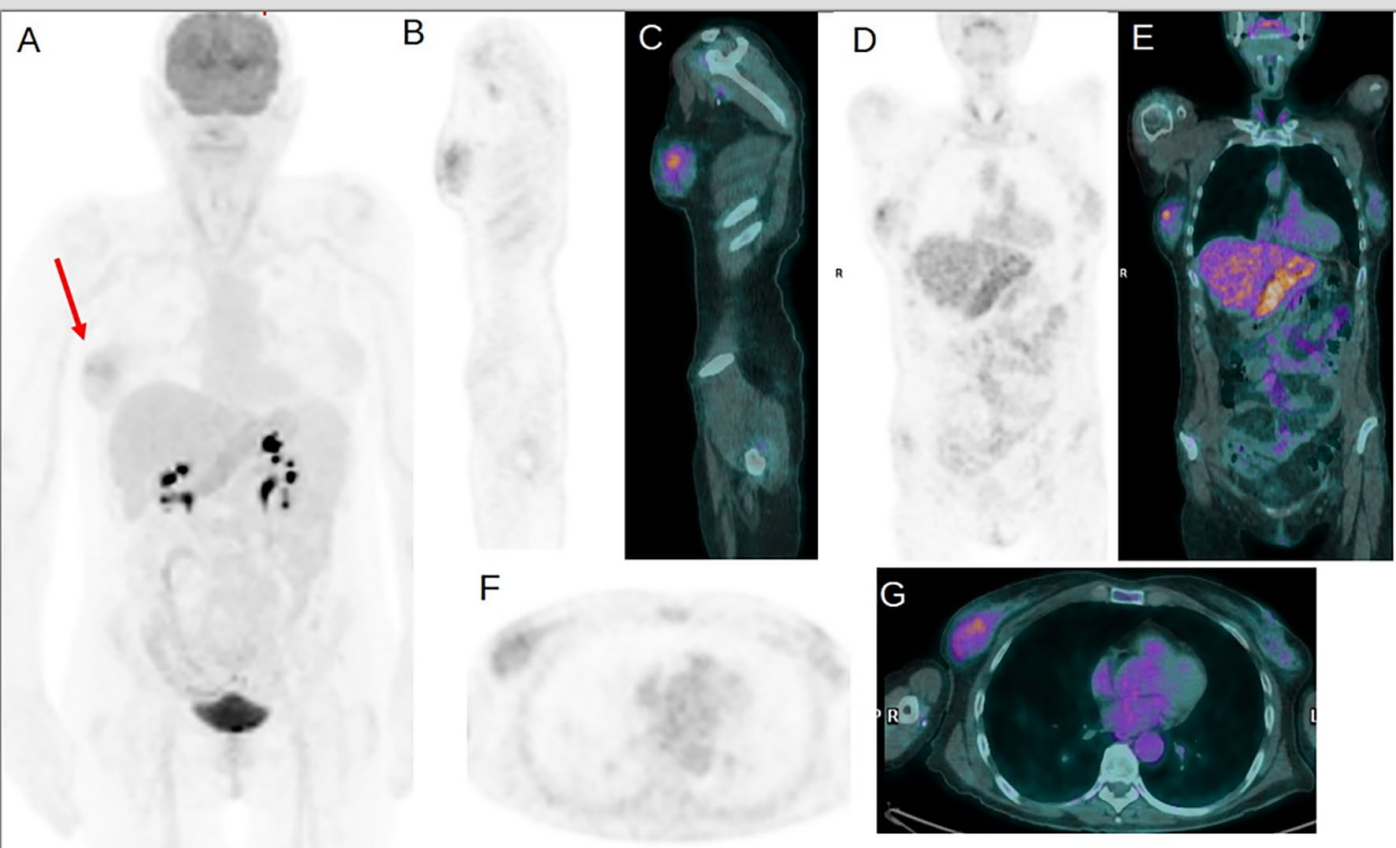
A 55-year-old woman was admitted to the hospital for subarachnoid hemorrhage and negative microbiological cultures, negative CT and echocardiography performed 2-[^18^F]FDG PET/CT for the search of any pathological uptake. Maximum intensity projection PET (A) revealed the presence of an increased uptake in the right breast (black arrow). Sagittal PET (B) and PET/CT (C), coronal PET (D) and PET/CT (E), axial PET (F) and PET/CT (G) confirmed that this focal uptake corresponded to a hypodense lesion with a central area of relatively reduced uptake on the right breast. This uptake was judged suspected for abscess. Subsequent breast biopsy demonstrated the benign nature of this lesion. The final diagnosis of this patient was encephalitis, but PET showed a physiological brain uptake without any area or reduced or increased uptake
